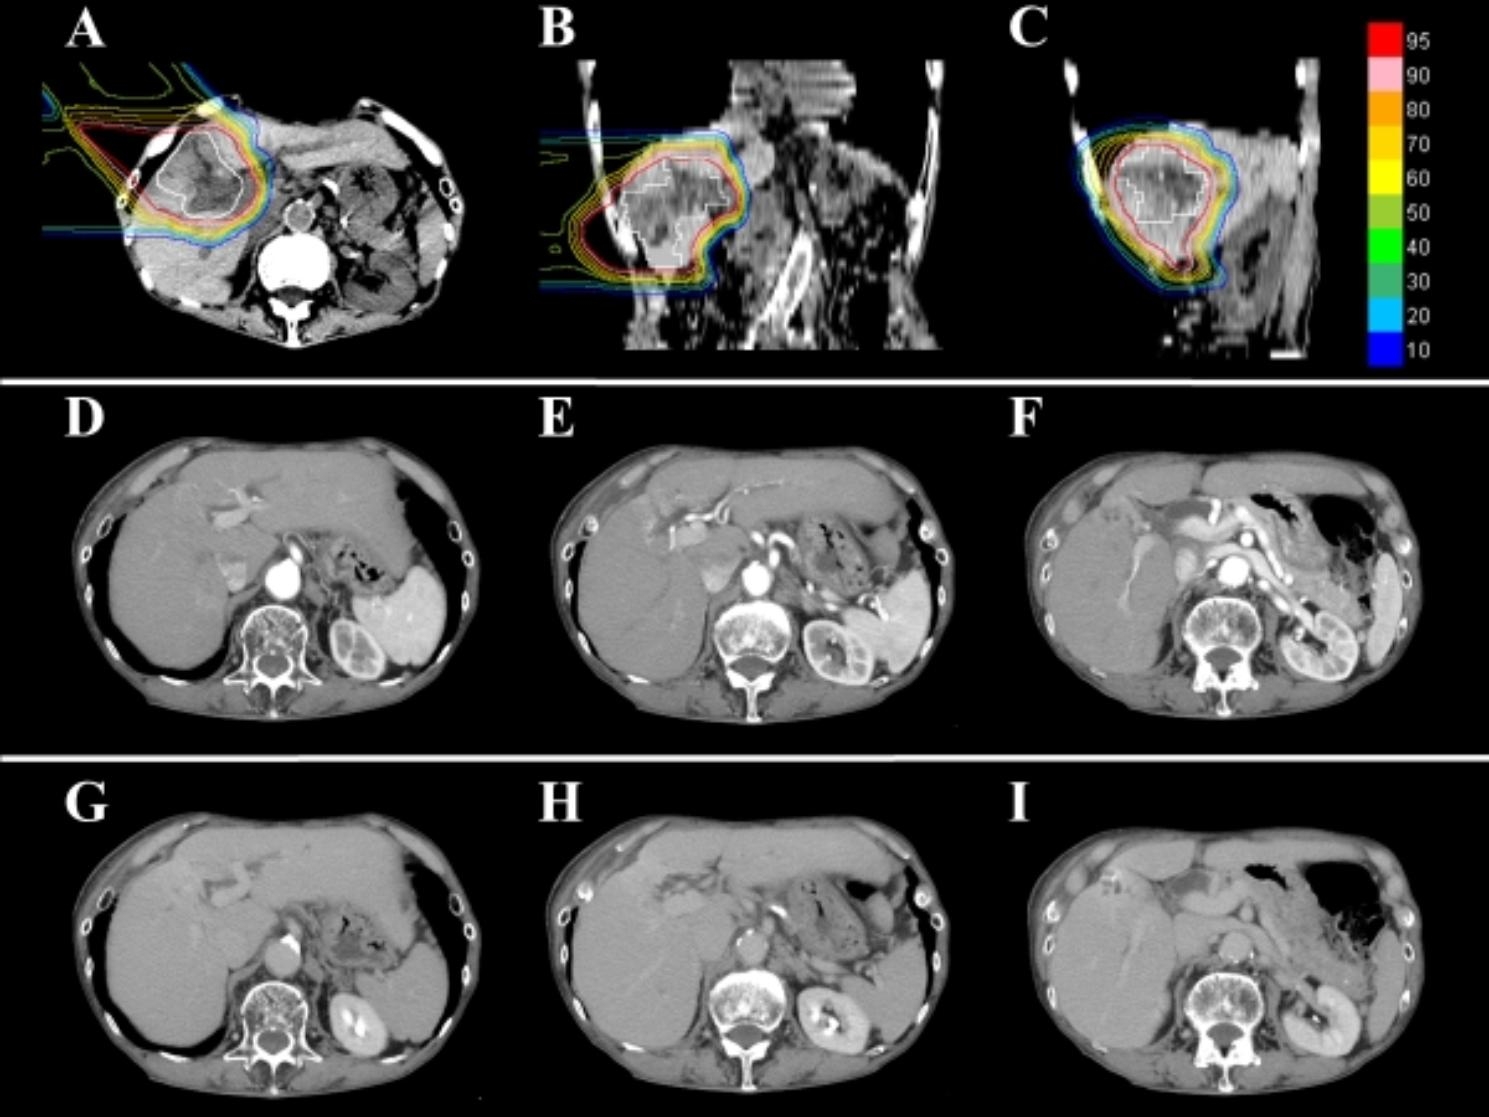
Pre-treatment computed tomography images of Case 1. (A-F) The arrowhead indicates bile duct tumor thrombus in axial slices. (G) The arrowhead indicates bile duct tumor thrombus in portal phase in a coronal slice. (H) The arrowhead indicates the location of bile duct tumor thrombus in magnetic resonance cholangiopancreatography
